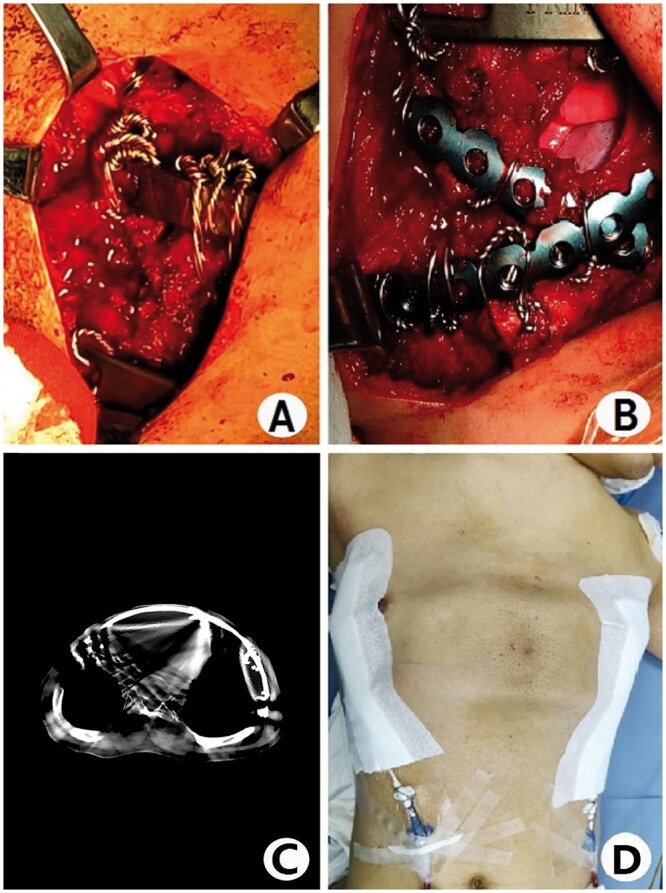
Perioperative and postoperative pictures. Operative pictures (A and B); postoperative chest computed tomography image (C); and postoperative appearance of the chest wall (D).
